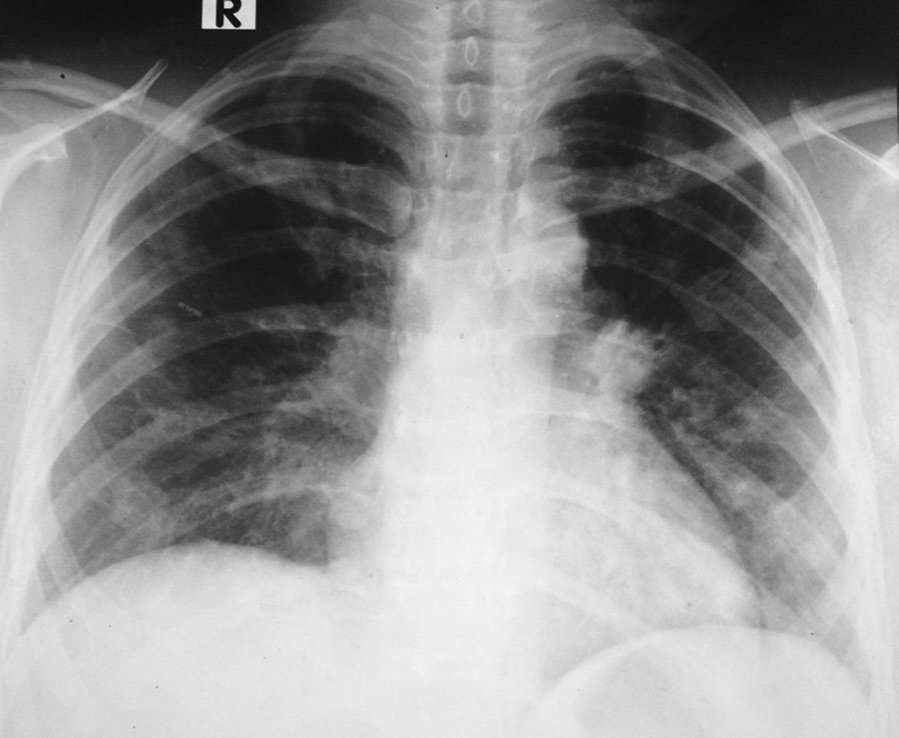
Chest radiograph with bilateral patchy shadows involving both lung fields
